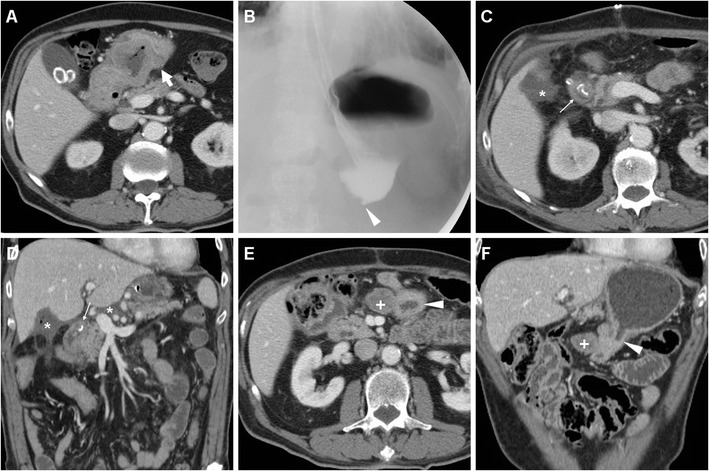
A 76-year-old male with stricturing antro-pyloric carcinoma (thick arrow) as preoperatively depicted by CT (A) underwent subtotal gastrectomy with Roux-en-Y reconstruction. Early postoperative CF (B) showed little or no transit through the GJS (arrowhead). On 8th POD during postoperative sepsis, contrast-enhanced CT (C, D) showed normal stapled DS (thin arrows), minimal fluid (*) in the cholecystectomy site. The patient then improved on conservative therapy including gastric intubation. Subsequent endoscopy showed ischaemic ulceration of the GJS. Repeated CT (E, F) three months later showed circumferential mural thickening of the GJS (arrowheads) and perianastomotic nodule (+) consistent with tumour recurrence
